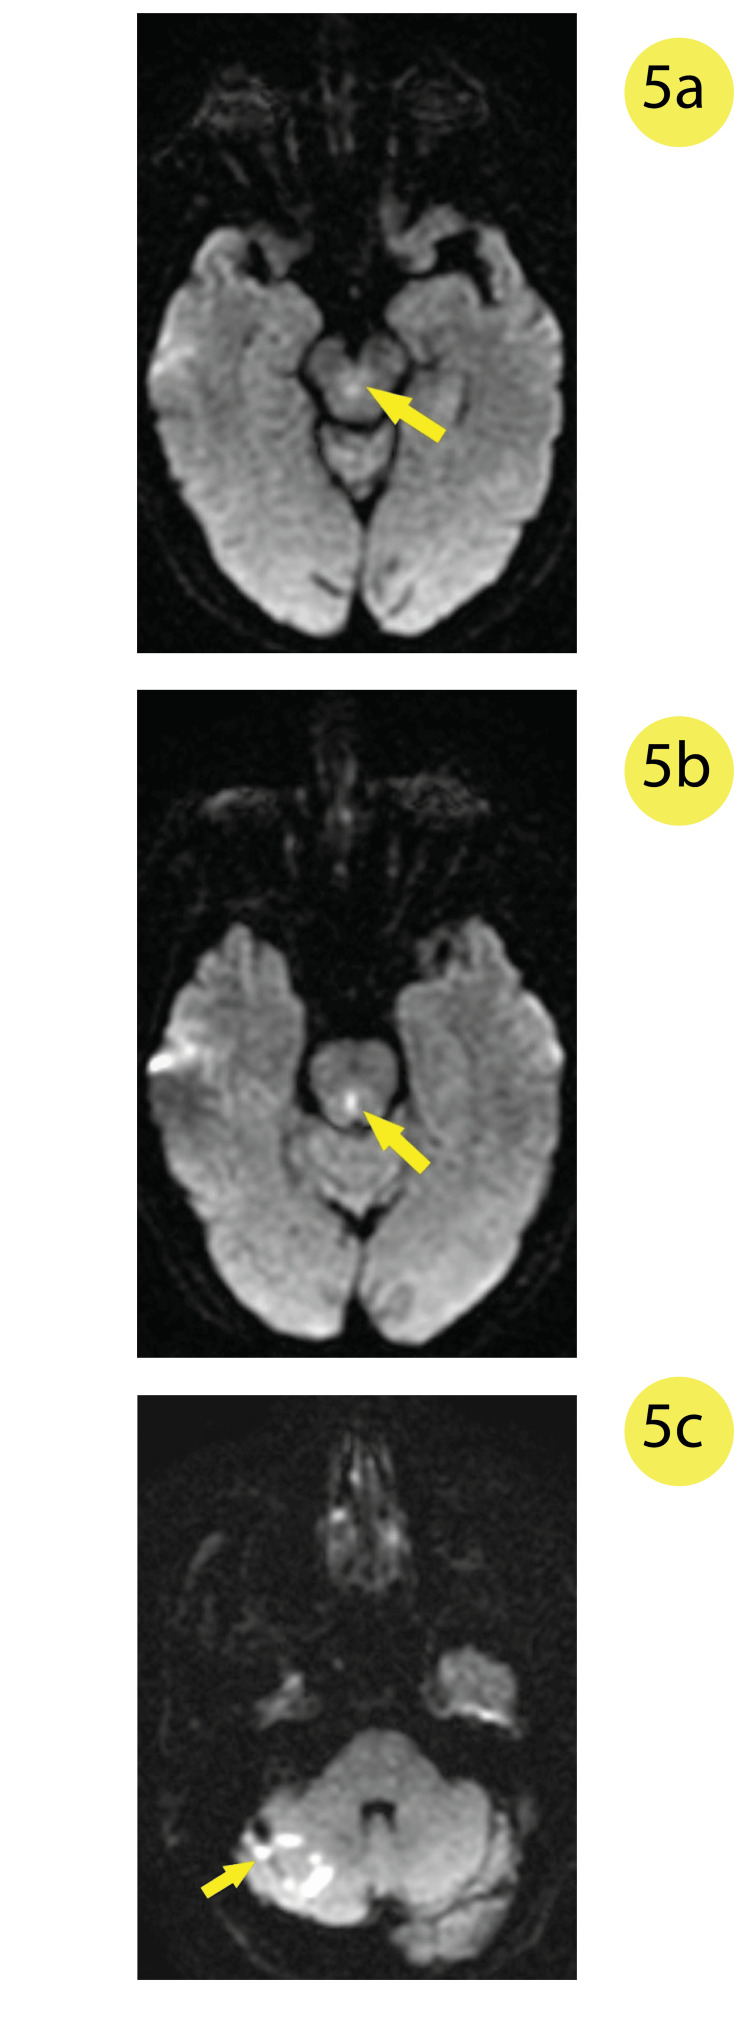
Diffusion-weighted image (DWI) MRI of the brain showing diffusion restriction in the a) central midbrain, b) central-posterior pons, and c) the right superior cerebellum
